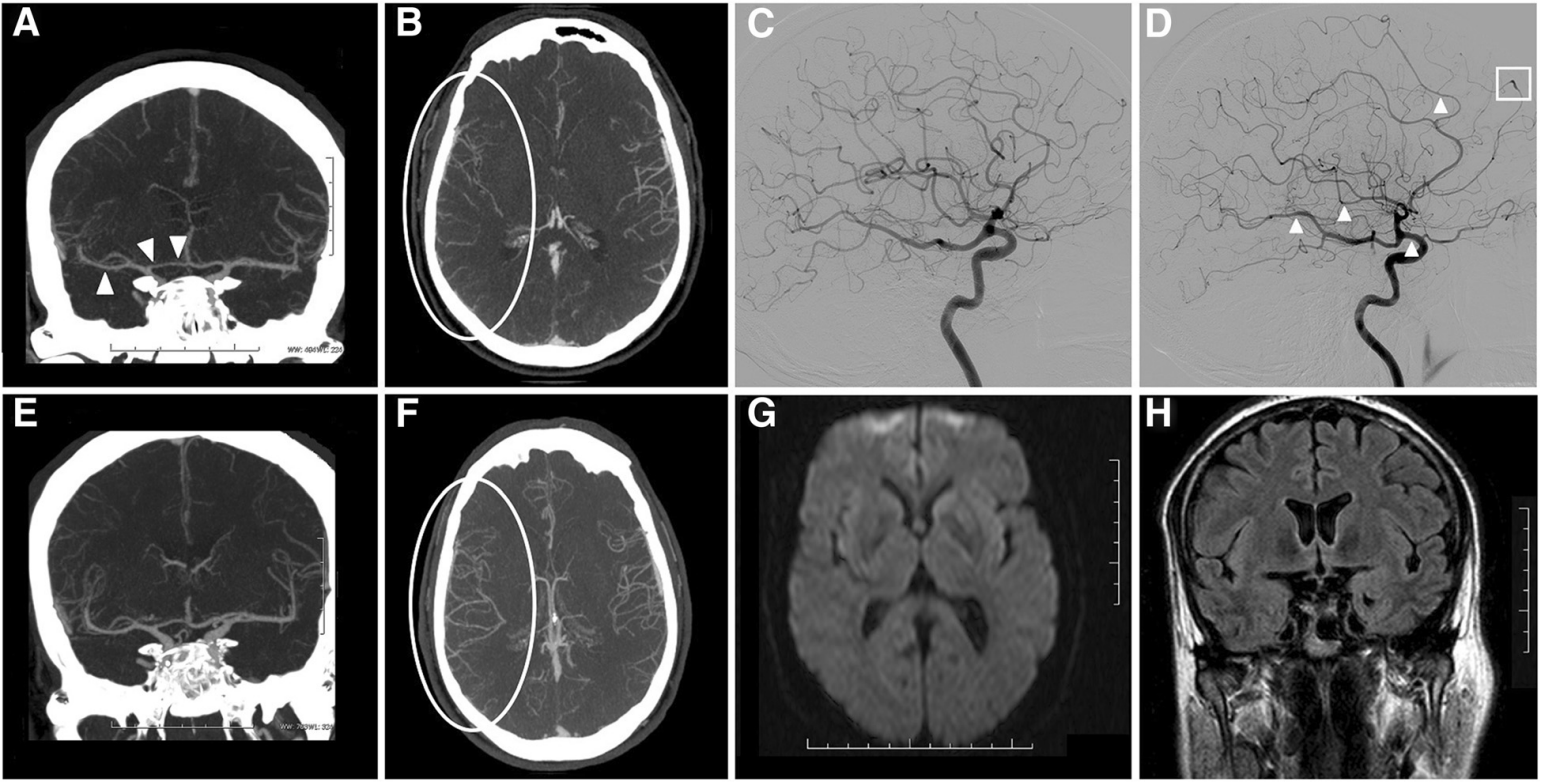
Vasospasm in cerebral arteries secondary to hypomagnesemia. Coronal (a) and axial (b) CT angiography showing subtle focal changes in the vascular lumen of the right carotid circulation (a, white arrows) and overall decrease of the vascularization in the territory of the right middle cerebral artery (b, circled in white). Selective cerebral angiography injecting from the left internal carotid artery (c), without abnormalities, and from the right internal carotid artery (d), showing overall decrease of the vascularization, focal narrowing (d, white arrows) and segmental stenosis of several arteries (d, white box). CT angiography carried out 7 days after the previous one (e and f) showing complete resolution of the vascular changes. Diffusion weighted imaging (DWI) (g) and fluid attenuation inversion recovery (FLAIR) (h) sequences of brain MRI showing no relevant abnormalities
